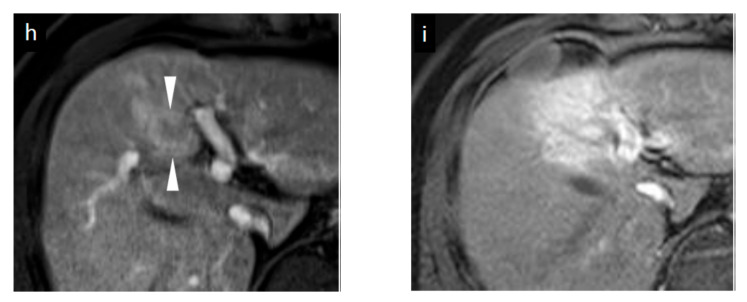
Grayscale ultrasound (US), contrast-enhanced US with Sonazoid (SCEUS), and contrast-enhanced MRI (CEMRI) images before and seven months after radiotherapy (RT) for a HCC lesion (maximum diameter: 22 mm) in segment IV, evaluated as no local recurrence after RT. A safe punctuation was difficult, as the lesion was located behind the gallbladder. The patient refused surgical resection, so they received RT. SCEUS before RT (a–c): A hypoechoic lesion was observed using grayscale US (a). This lesion showed hypervascularity during the arterial phase (AP) of SCEUS (b) and hypoechoic (perfusion defect) during the post vascular phase (PVP) (c). SCEUS seven months after RT (d–g): Grayscale US showed a hypoechoic nodule and a reduction in tumor size (d). AP SCEUS showed a disappearance of vascularity of the HCC lesion and hypervascularity of the surrounding liver parenchyma (e). Both the HCC lesion and the surrounding liver parenchyma appeared as a perfusion defect during the PVP (f). After re-injection of Sonazoid during the PVP, the HCC showed hypovascularity and the surrounding liver parenchyma showed hypervascularity (g). AP CEMRI before (h) and seven months (i) after RT: Before RT, this lesion showed hyperintensity during AP CEMRI (h). Both the HCC lesion and the surrounding liver parenchyma appeared as hyperintensity at seven months after RT (i). Seven months after RT, we observed a discrepancy between the vascularity shown by SCEUS and that by CEMRI, but the lesion has not recurred for more than 3 years, suggesting that the SCEUS findings might be correct. Arrowheads show the margins of the HCC lesion. Arrows show the margins of irradiated surrounding liver parenchyma.
